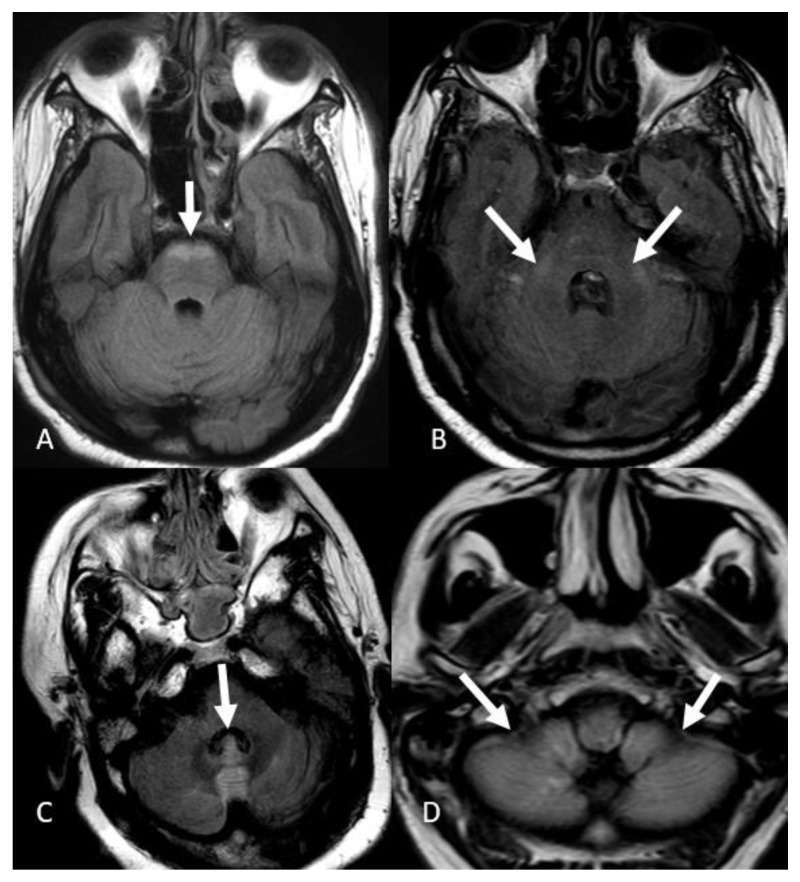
Posterior fossa involvement. (A) Axial FLAIR images demonstrate FLAIR hyperintensity in the ventral portion of the pons. (B) Bilateral middle cerebellar peduncle FLAIR hyperintensities. (C) FLAIR hyperintensity of the vermis. (D) FLAIR hyperintensities in the bilateral cerebellar hemispheres. Image has been windowed manually to improve the visibility of signal abnormality.
